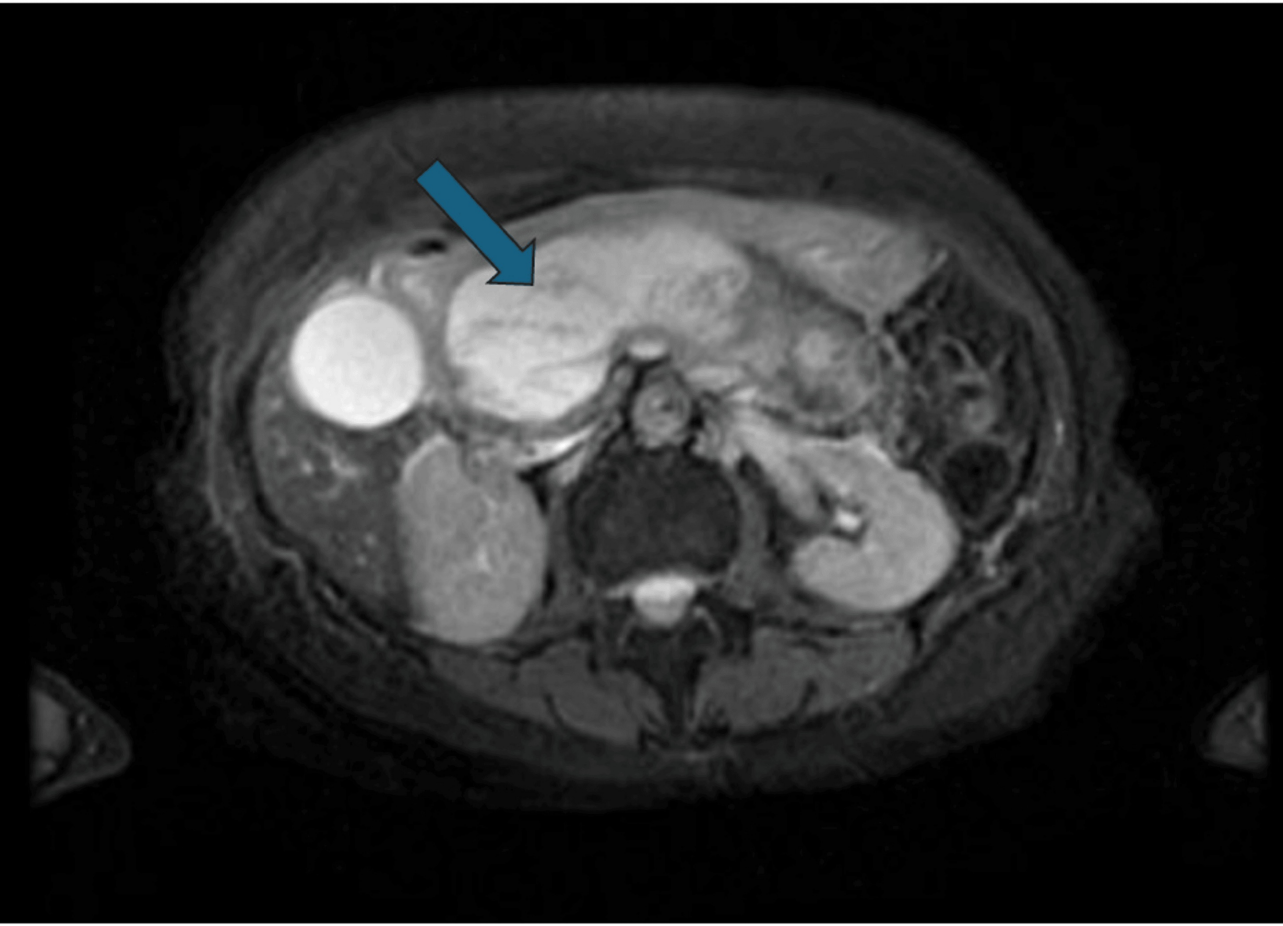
Walled-off fluid collection in the pancreas. Abdominal MRI showing a walled-off 14 cm pancreatic fluid collection, obstructing the common bile duct.
